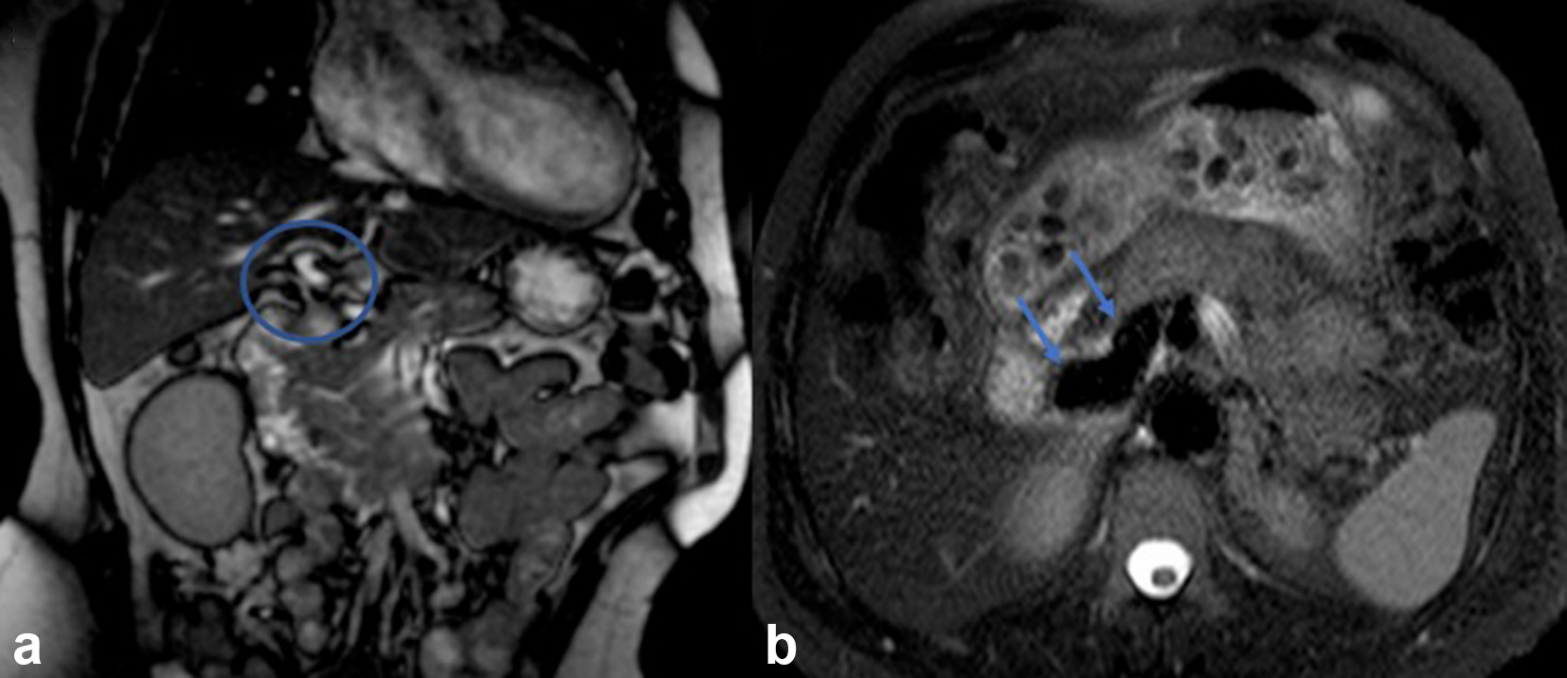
MR images show the presence of the shunt with the inferior vena cava (arrows in b), and abnormal portal vein (circle in a).
